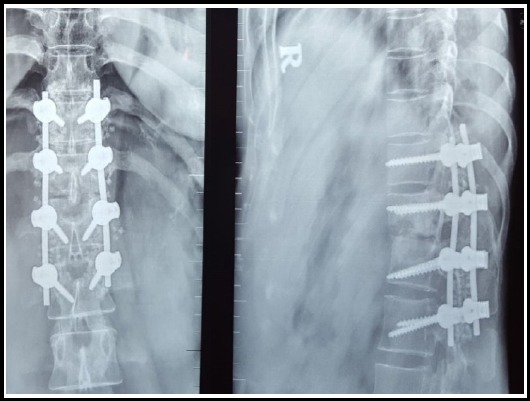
Plain X-ray thoracolumbar spine showing post-operative images of a D11-L2 fixation for a D12-L1 collapse.
